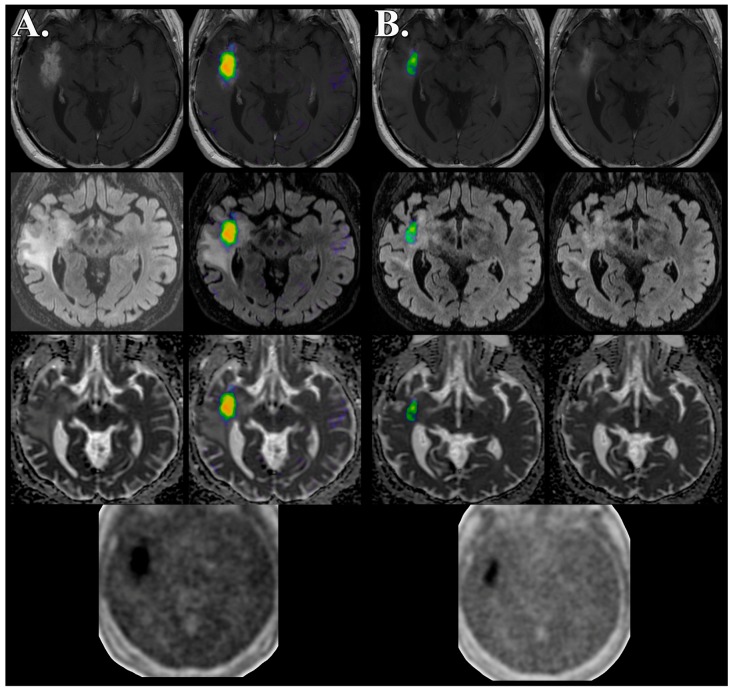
Decreased but persistent FMISO hypoxic volume in recurrent high grade glioma concurrent with bevacizumab therapy. FMISO PET/MR imaging obtained simultaneously 7 days prior to (A) and 36 days following antiangiogenesis therapy (B) demonstrates response to therapy characterized by decreased volume of contrast enhancement (top row) and nonenhancing FLAIR hyperintense mass (middle row) without evidence of reduced diffusion (adc map, bottom row). Unprocessed (bottom) and fused FMISO PET imaging (baseline, middle left; follow-up, middle right) demonstrates decreased but persistent tumor hypoxic volume predominately within the enhancing component. Follow-up MR imaging did not demonstrate the development of nonenhancing reduced diffusion mass at any point of therapy.
